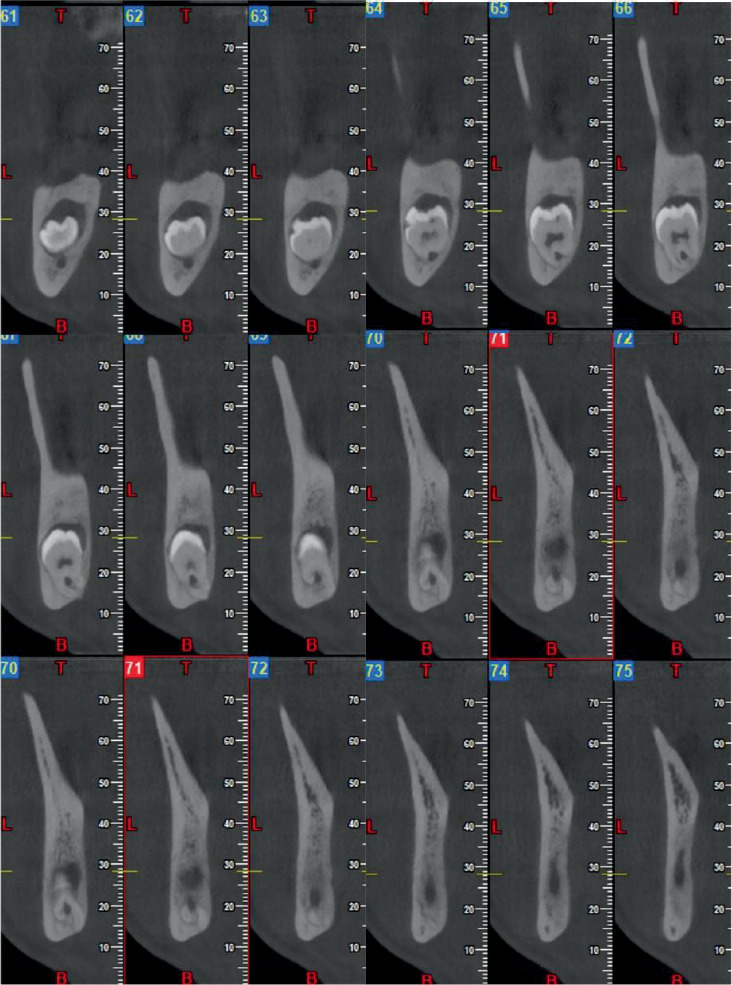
Preoperative cone beam computed tomography. The inferior alveolar nerve was trapped in the furcation roots of the lower third molar.
